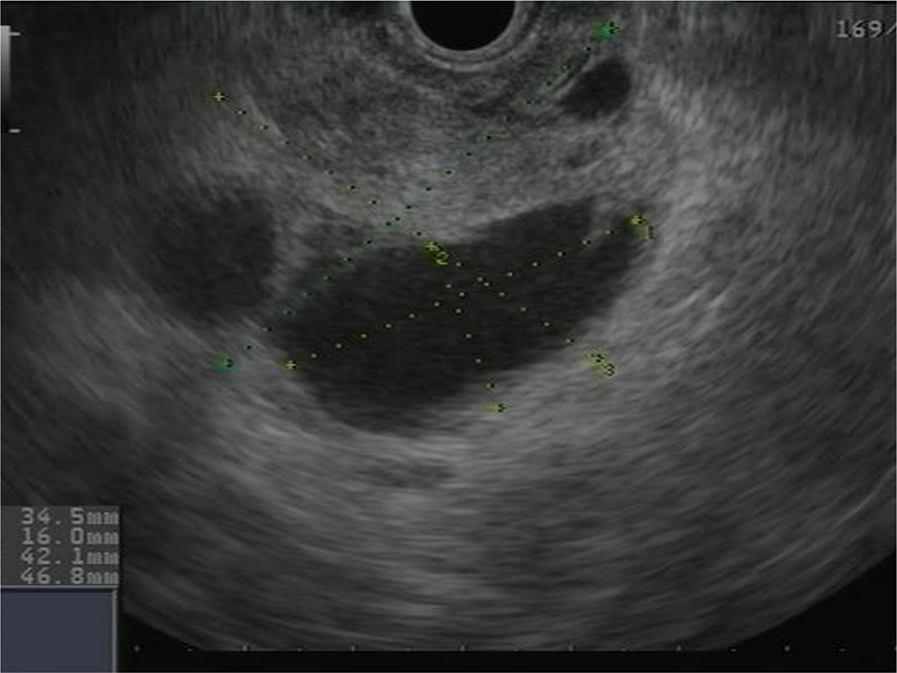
Endoscopic ultrasound image revealing a cystic lesion with regular well-demarked borders in the head of pancreas.
